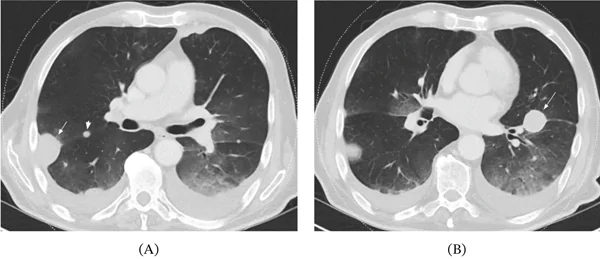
Chest computed tomography (CT) 6 days after admission. (A) Newly developed nodules in the right lung (arrowhead indicates one of the new nodules), together with progression of previously identified lesions (arrow). (B) Marked progression of a nodule along the left interlobar fissure (arrow). Small bilateral pleural effusions are also present.
